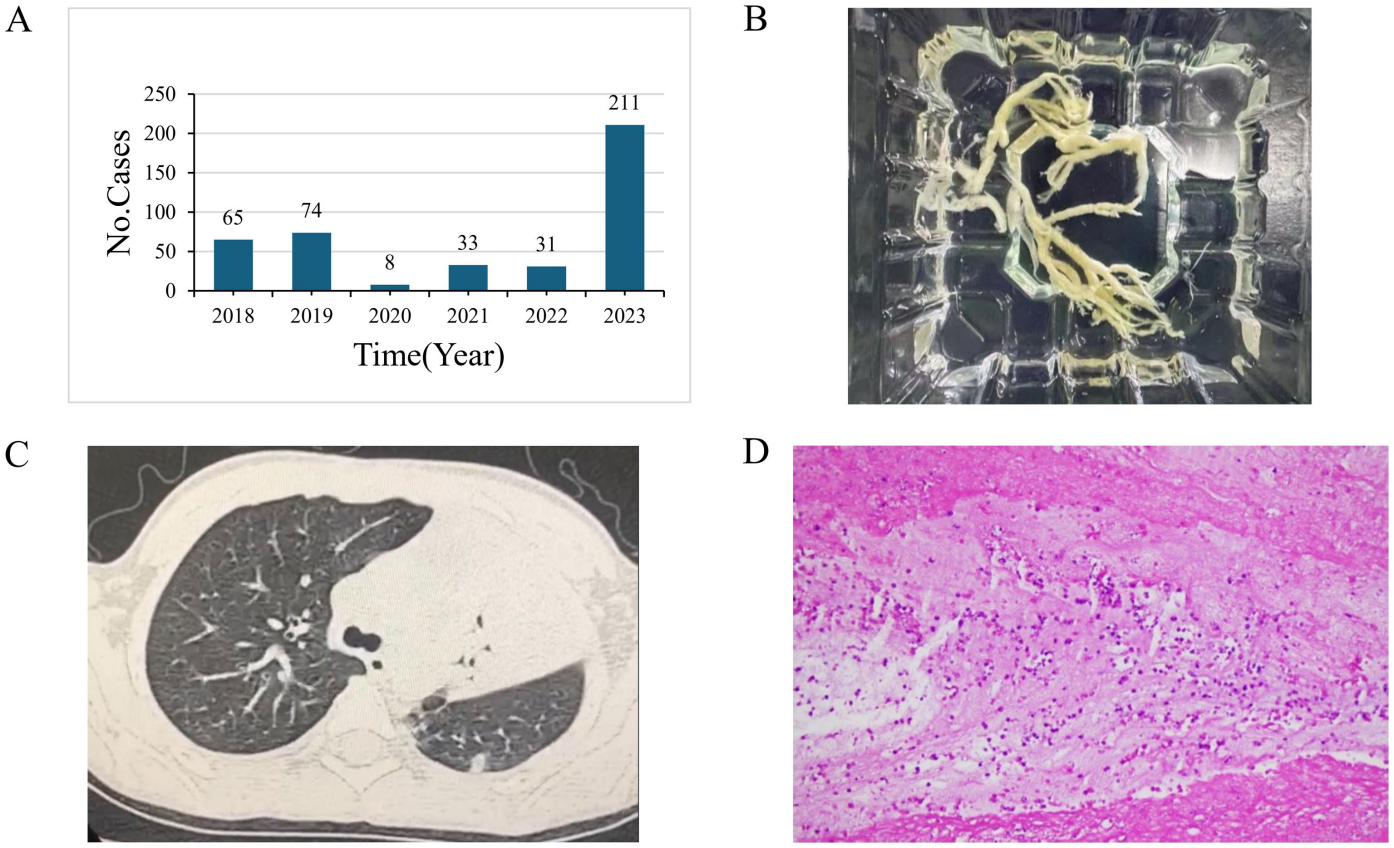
(A) The number of Plastic bronchitis (PB) cases from 2018 to 2023. (B) A tree-shaped sputum plug post-removal. (C) Chest CT findings in patients diagnosed with PB. (D) Pathological image from a tracheoscopic airway mucosal biopsy of a patient with PB, showing fibrin-like inflammatory exudates and a locally high lymphocytic and neutrophilic infiltrate.
